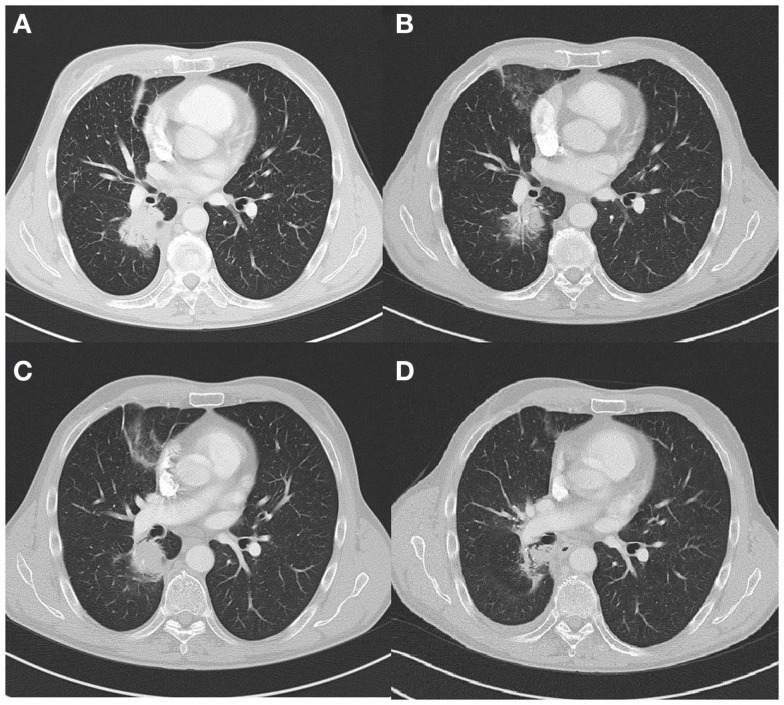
(A) Tumor lower right lobe on diagnosis (CT scan). (B) Partial response 22 months after start TKI (CT scan). (C) Local progression 26 months after diagnosis (CT scan). (D) Partial response 9 months after local radiotherapy (CT scan).
